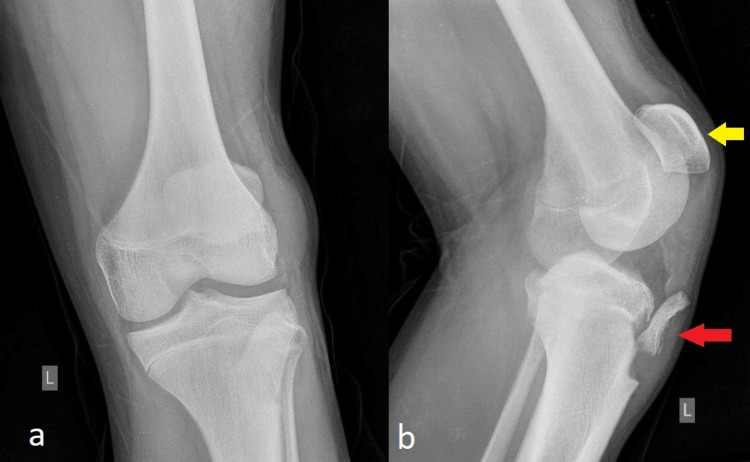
Anteroposterior (a) and lateral (b) radiographs of the injured knee Avulsion fracture of the tibia tubercle (red arrow) and high-riding patella (patella alta; yellow arrow) are noted.
